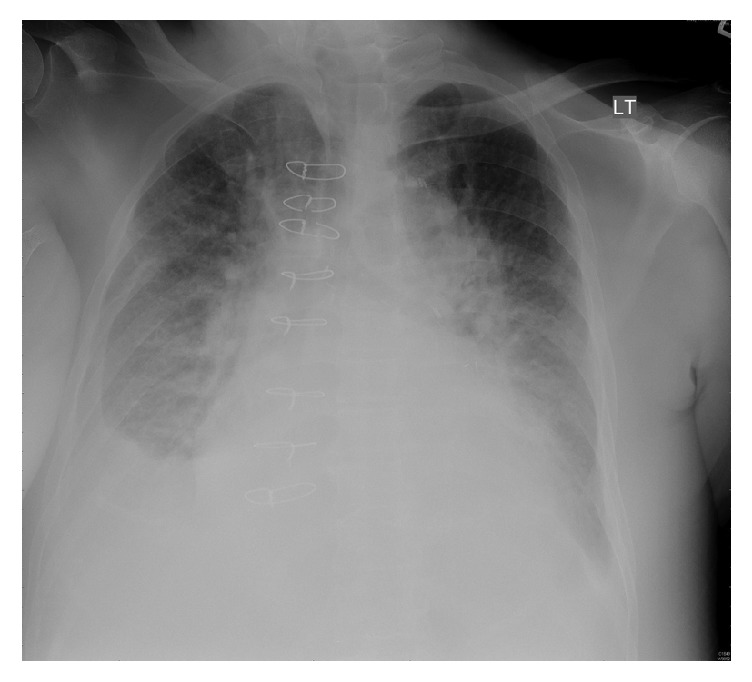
Portable chest radiograph on presentation showing bilateral basilar opacities with pleural effusions and hilar congestion.
